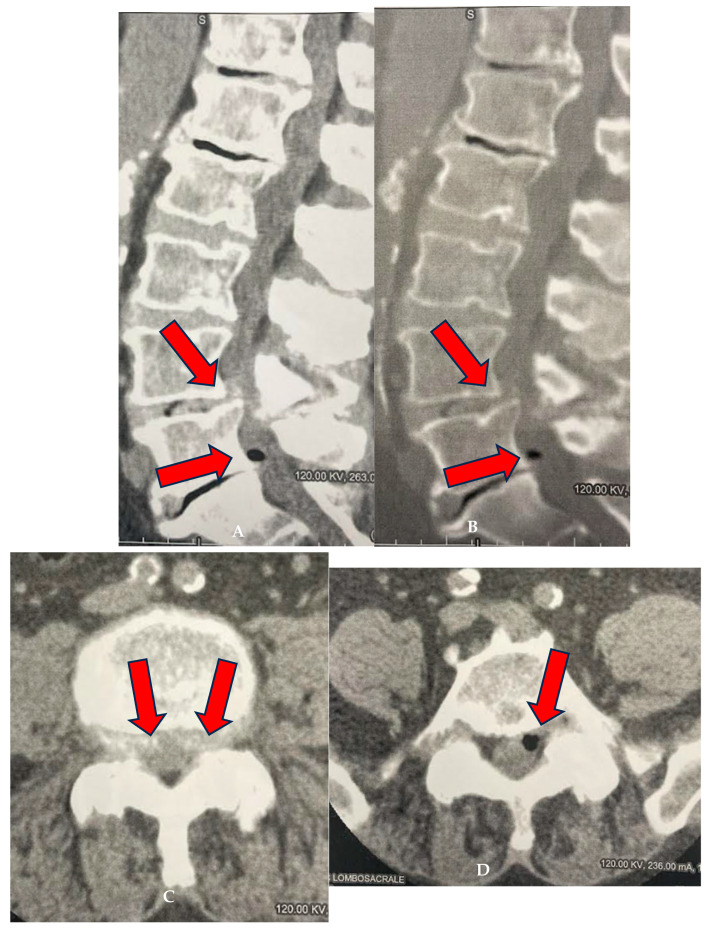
(A–D): FG of 77-year-old male treated at L4–L5 and L5–S1 levels. (A,B) Sagittal CT reconstructions using algorithms for both parenchyma and bone. L4–L5 spondylolisthesis (arrows), degenerated and thinned L5–S1 disc with vacuolated left paramedian herniation (arrow). (C) Axial CT scan at L4–L5: bilateral paramedian central protrusion of the annulus (arrows). (D) Axial scan at L5–S1: partially vacuolated left paramedian herniation causing indentation on the emergence of the left S1 nerve root (arrow).
